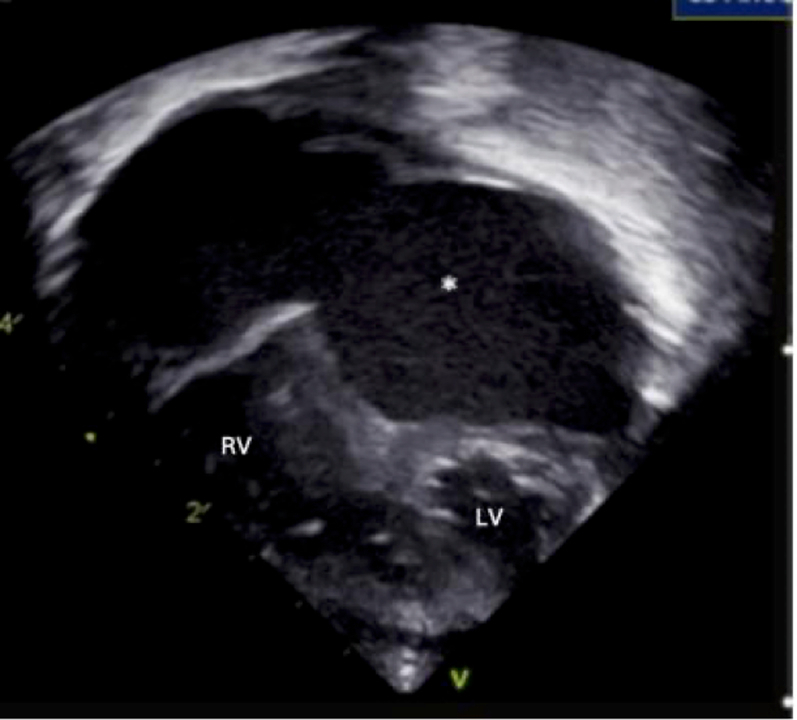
Postnatal Transthoracic Echocardiogram Showing Large Coronary Sinus Aneurysm (Asterisk) Along the Left Posterior Atrioventricular Groove LV = left ventricle; RV = right ventricle.
